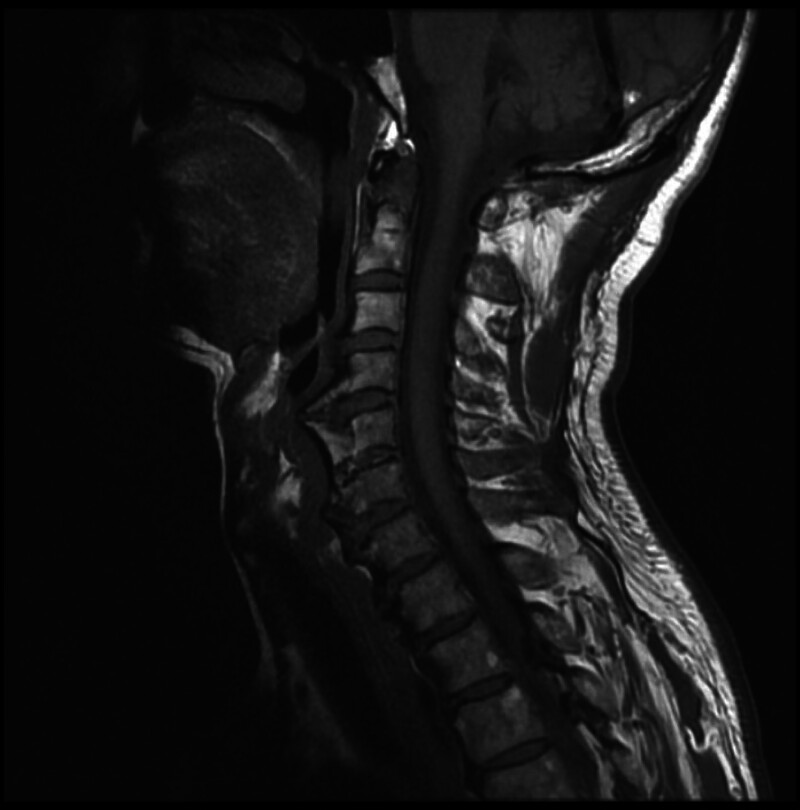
Preoperative T2-weighted MRI of the cervical spine demonstrating prominent anterior osteophytes at C4 to C7, causing compression and displacement of the esophagus. MRI = magnetic resonance imaging.
